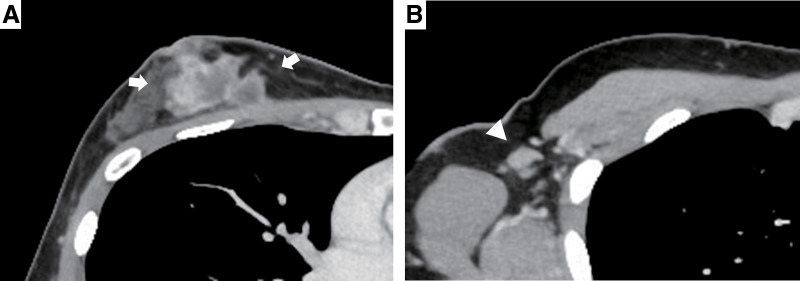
Contrast-enhanced CT images at presentation revealed a 4.3 × 4.3-cm tumor in the upper-inner quadrant of the right breast (A, arrows), with enlarged axillary lymph nodes (B, arrowhead).
